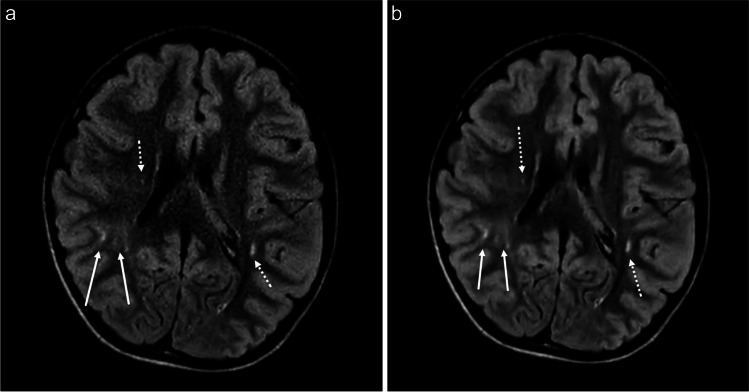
a-b Axial T2W FLAIR CS=1.6 brain MR in a 8-year-old male with known diagnosis of tuberous sclerosis with standard (a) and AI (b) reconstruction demonstrating a dysplastic lesion in the right parietotemporal junction involving the subcortical and transmantle white matter (solid white arrows). There are additional smaller T2W FLAIR hyperintense foci in the bilateral periventricular white matter (dashed arrows), also accurately reproduced on AI reconstruction
